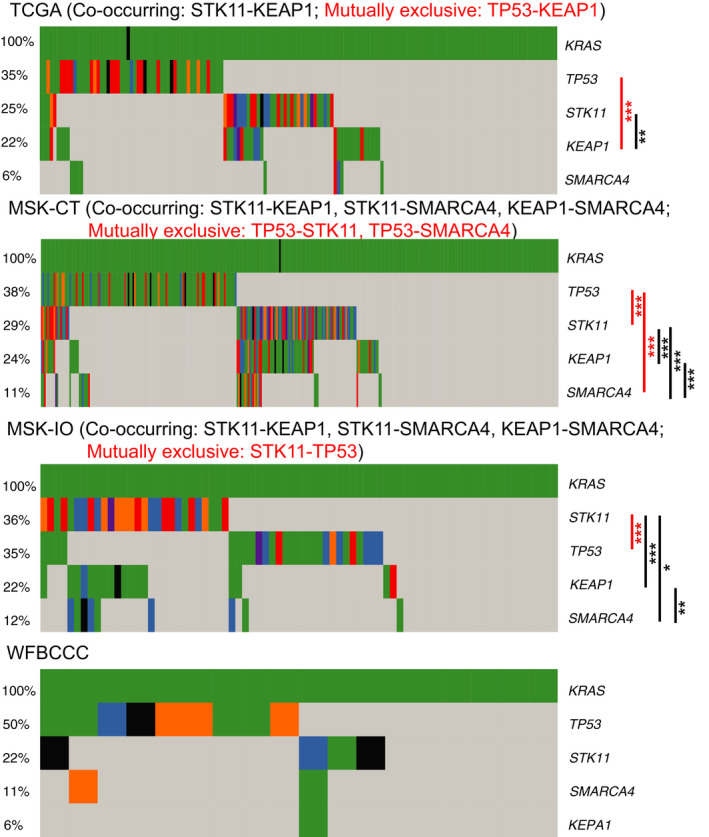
Global somatic mutation landscape of *KRAS*, *TP53, STK11, KEAP1, and SMARCA4* genes in the TCGA, MSK‐CT, MSK‐IO, and WFBCCC cohorts. Comutations were determined with Fisher’s exact test. Black *,,: *P *< 0.05, 0.01, 0.001 for co‐occurrence; red *: *P *< 0.001 for exclusive occurrence.
